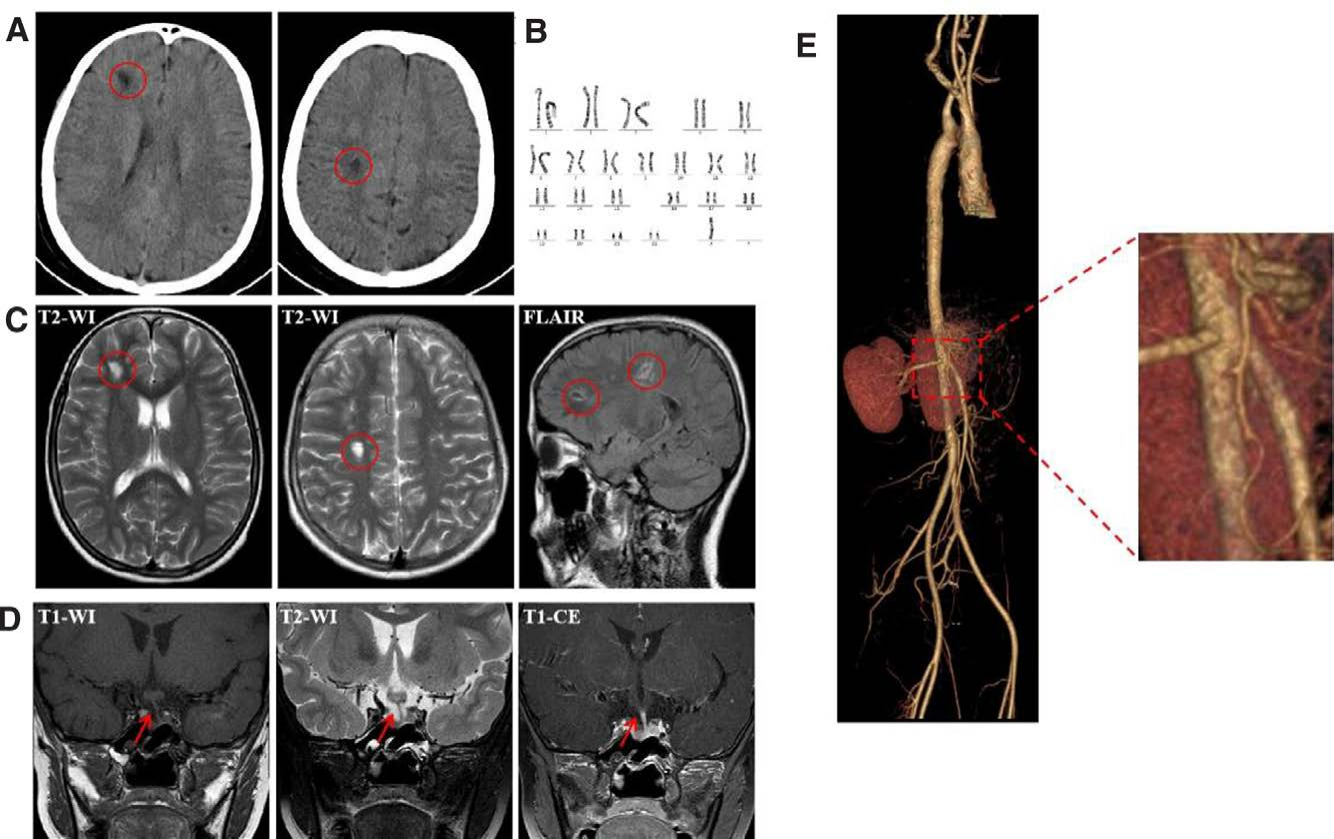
Imaging data and chromosome karyotype analysis. (A) Head CT, red circle marked low-density focus in the right frontal lobe and radiative crown; (B) chromosome karyotype analysis showed complete monosomy X (45, X); (C) brain MRI, red circle marked multiple abnormal signals in the right frontal lobe and radiative crown; (D) pituitary gland MRI, red arrow marked the pituitary stalk deviated to the left; (E) total aortic contrast-enhanced CT, the angle between the superior mesenteric artery and the abdominal aorta was 34.7°, without stenosis or occlusion. CT = computed tomography, MRI = magnetic resonance imaging.
